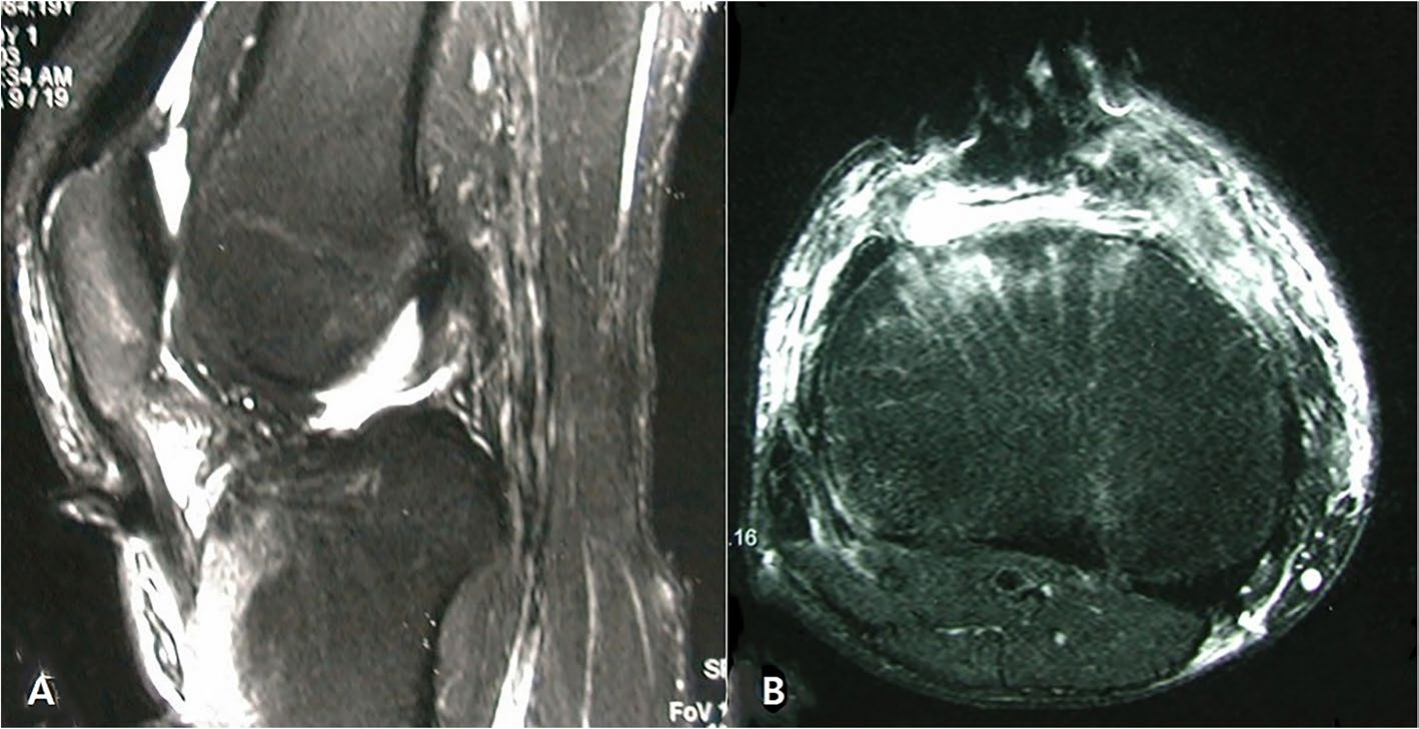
Initial T2-weighted MRI images showing patellar tendon rupture observed as defect on the distal part. a Sagittal image. b Axial image
